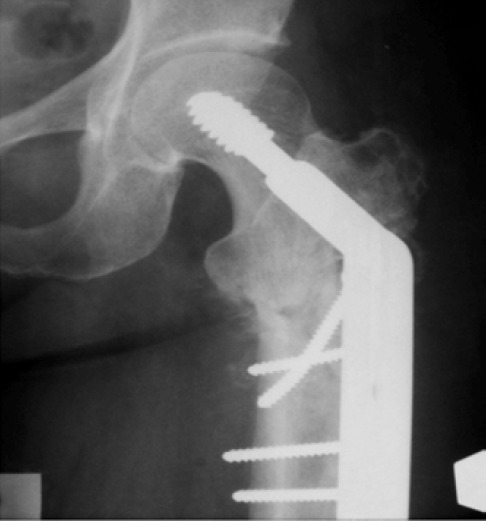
Radiograph taken two months postoperatively showing healing callus formation
